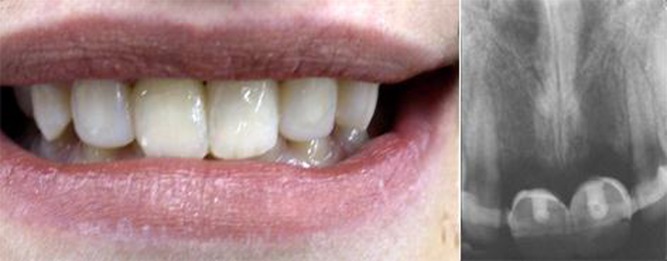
Clinical and radiograph aspects of the adhesive prosthesis
